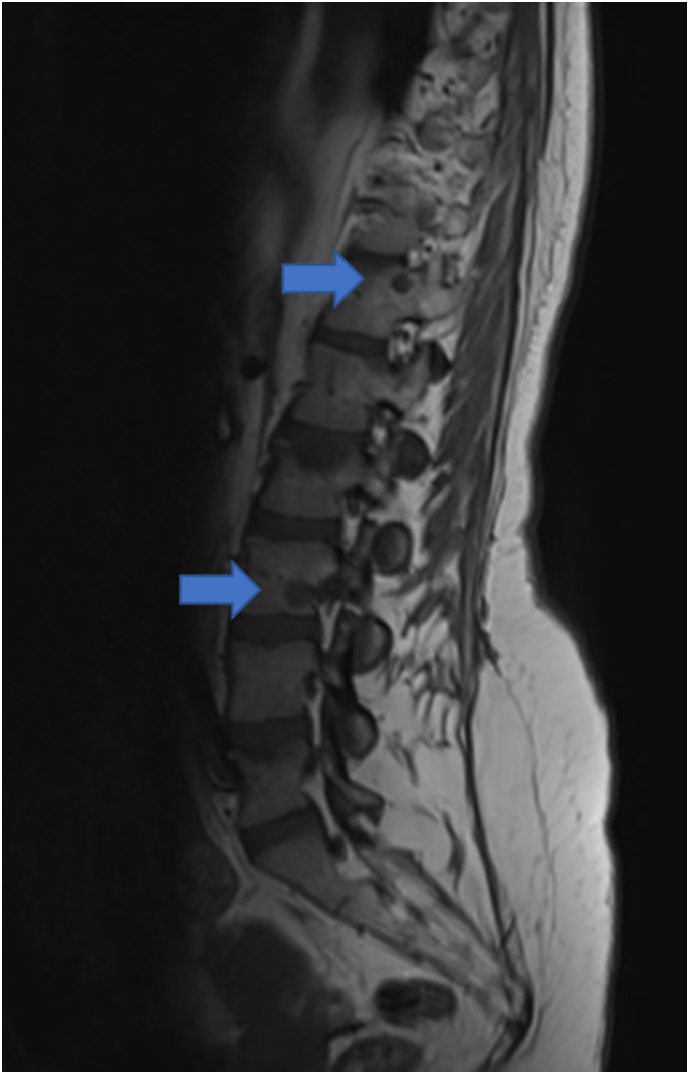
MRI T1-image of the lumbar spine April 2014. Blue arrows indicate T1 hypo-intense metastatic lesions at peduncle of Th12 and corpus of vertebra L3.
